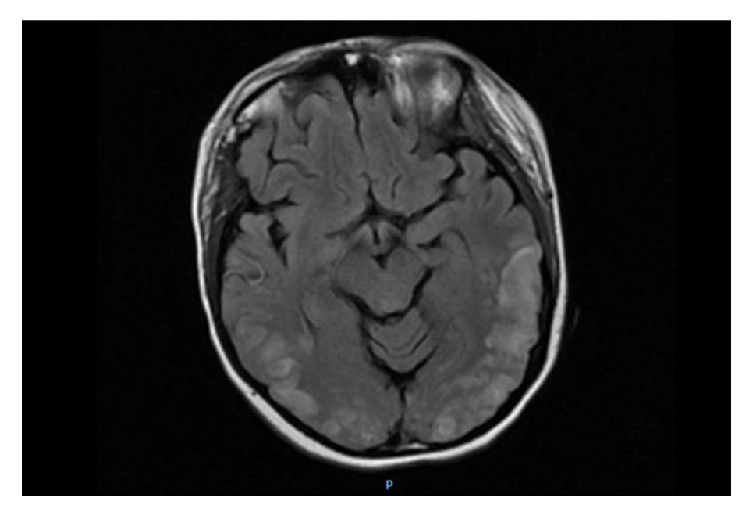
Brain magnetic resonance imaging (MRI) demonstrated symmetrical bilateral hyperintensities on T2-weighted imaging and fluid-attenuated inversion recovery (FLAIR) sequences consistent with cerebrocortical edema.
